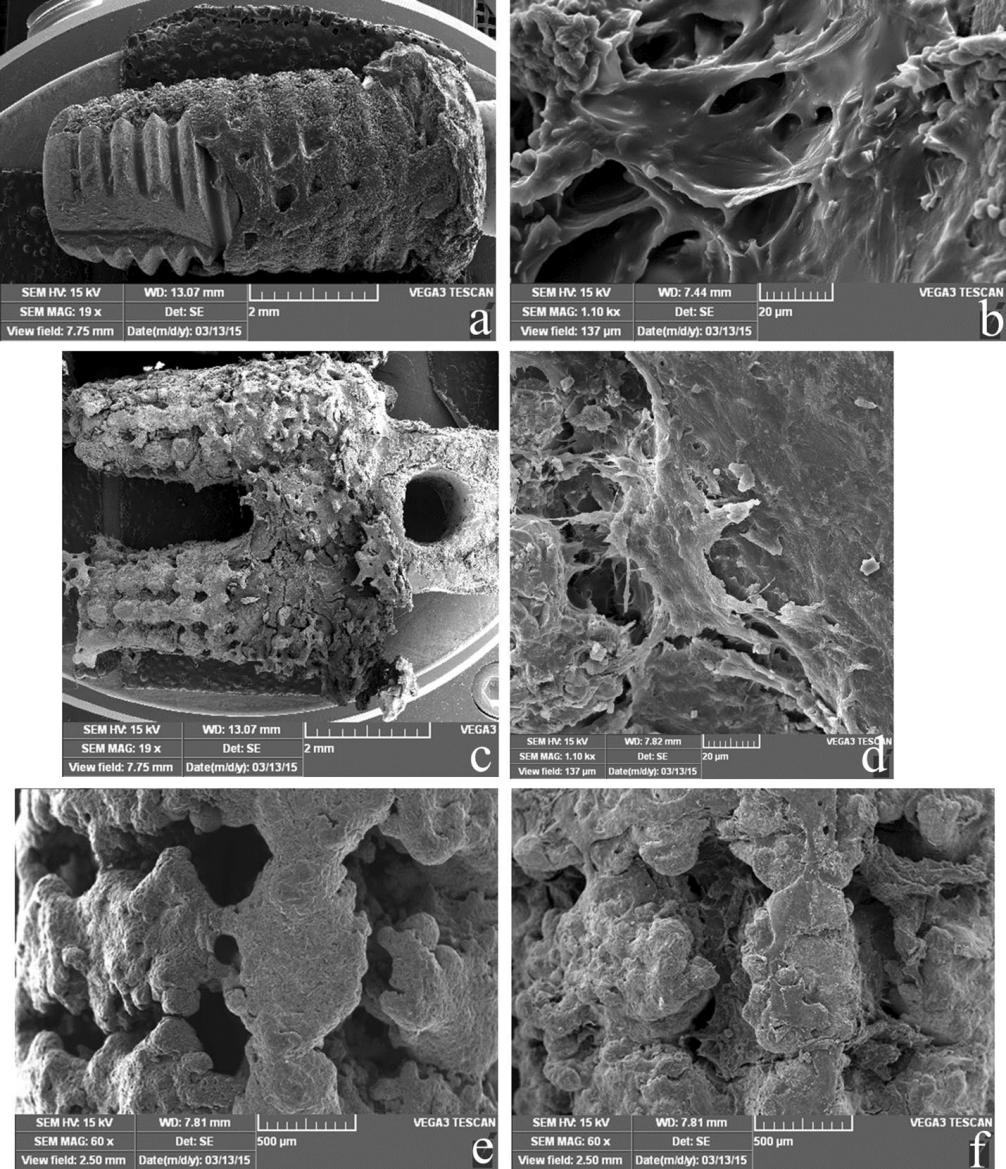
SEM images of the push-out implants 8 weeks after implantation. a A global image of an RBM implant. b A high-resolution image (×1.1 K) of an RBM implant. c A global image of an MRI. d A high-resolution image (×1.1 K) of an MRI. e An image of the cancellous part of an MRI before implantation. f An image of the part at (e) 8 weeks after implantation
